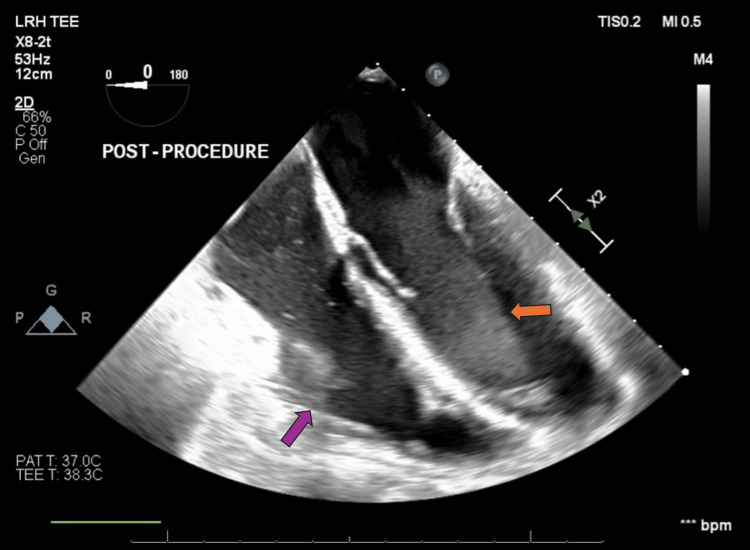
Transesophageal echocardiogram post-pericardiectomy (mid-esophageal four-chamber view) The purple arrow points to the right ventricle, now exhibiting normal size and filling, indicating the resolution of the previous compression. The orange arrow points at the left ventricle, also showing improved contour, suggesting restored ventricular dynamics post-procedure. These findings suggest successful alleviation of constrictive physiology.
